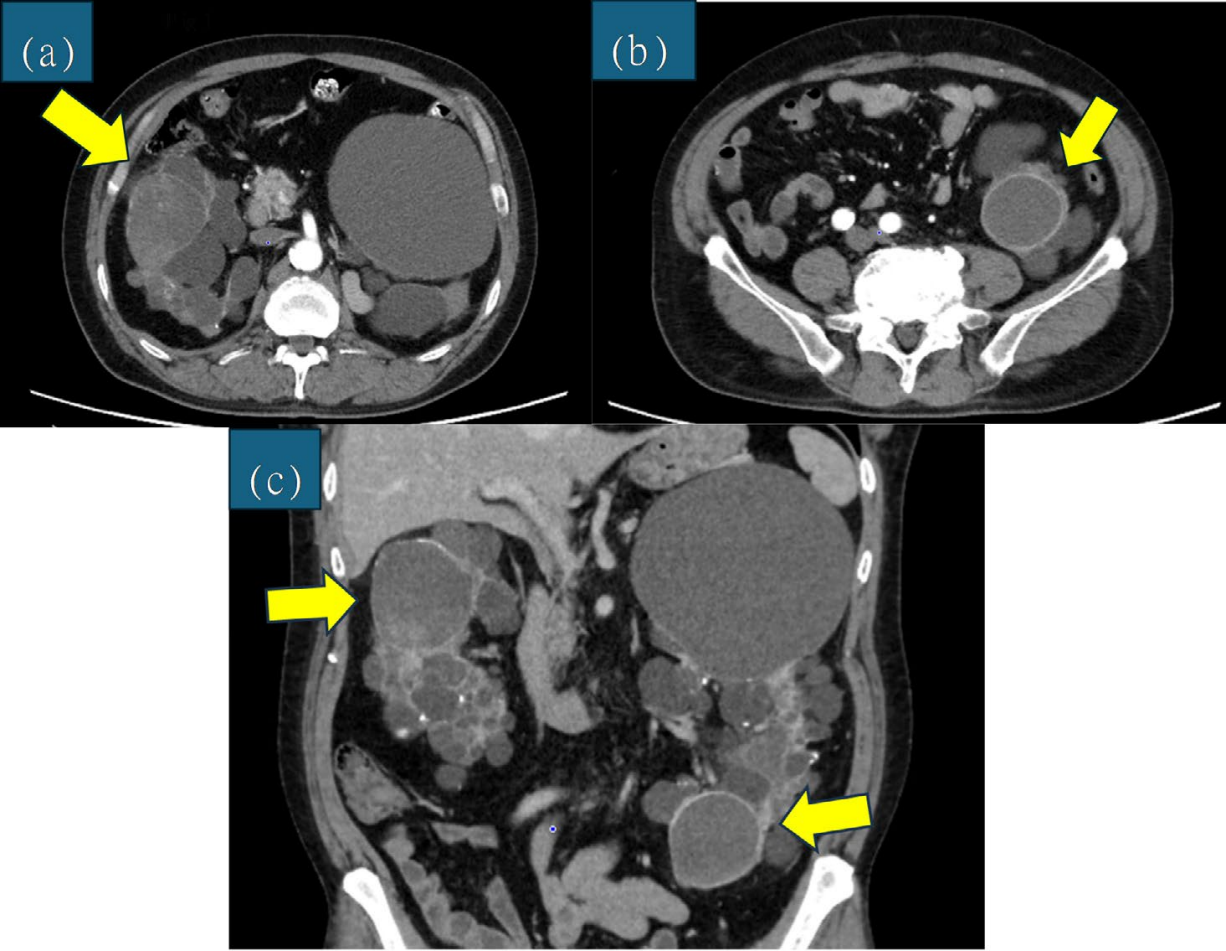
Contrast‐enhanced CT images. (a) Right kidney cancer, axial, (b) left kidney cancer, axial, (c) coronal. Cystic renal masses with a tendency to enlarge were observed in the mid‐pole of the right kidney and the lower pole of the left kidney, each containing an enhancing nodule. The maximal diameters were 64 and 49 mm, respectively. No obvious metastasis or venous invasion was detected. Right kidney dimensions: 133.5 mm (length) × 69.6 mm (width) × 146.3 mm (height). Left kidney dimensions: 170.0 mm (length) × 123.8 mm (width) × 234.3 mm (height).
